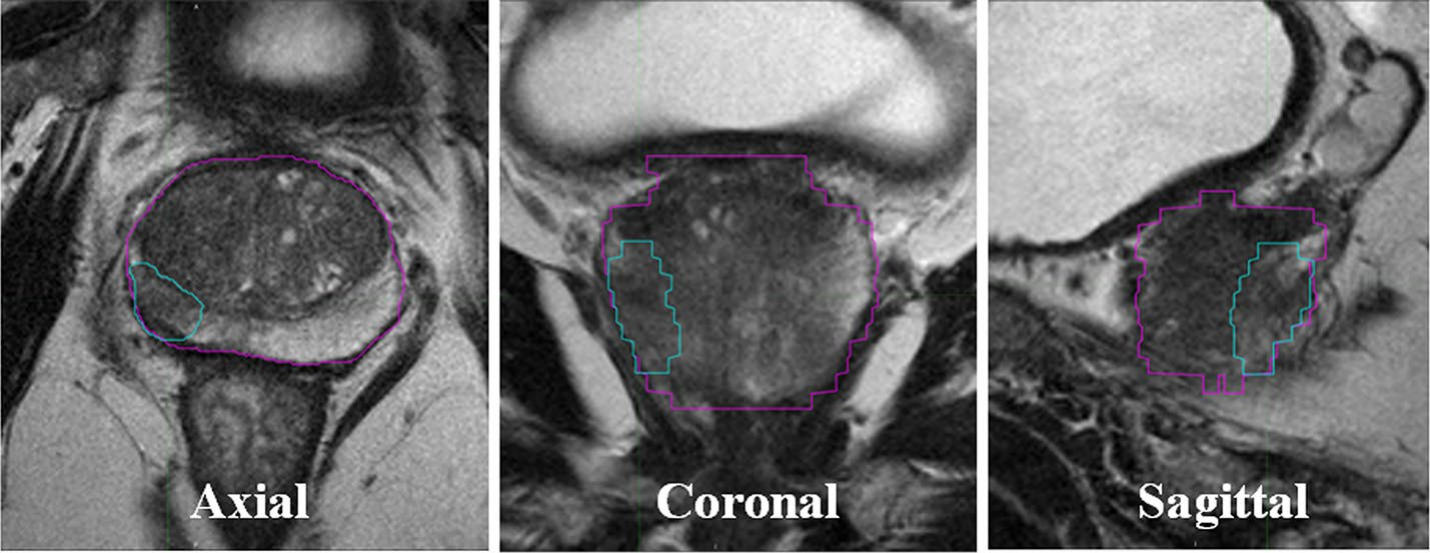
Multiplanar T2-weighted fast-spin echo (T2W-FSE) images (axial, coronal, and sagittal midplanes) of a patient with localized prostate cancer treated with EBRT (45 Gy/25), followed by an HDR BT boost (15 Gy/1). The prostate (*purple*) and dominant intraprostatic lesion (*light blue*) are not readily visible without supplementary functional imaging
